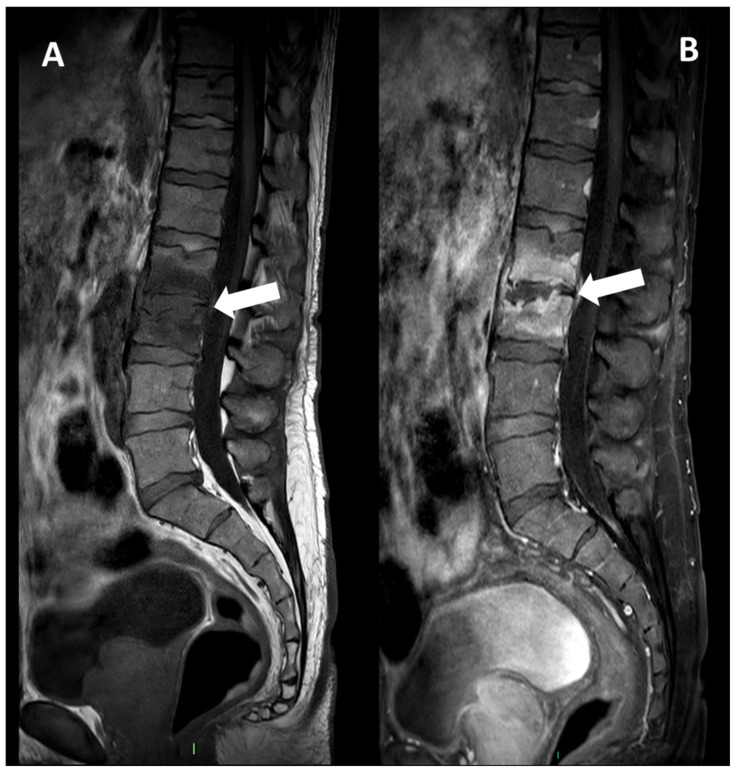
MRI, Sagittal T1w (Panel A), and T1w after contrast media injection (Panel B) of a 54-year-old male with pyogenic spondylodiscitis of L2-L3 vertebrae: complete alteration of disc signal intensity, endplates erosions, and diffuse pattern of vertebral body enhancement are detectable (arrows).
